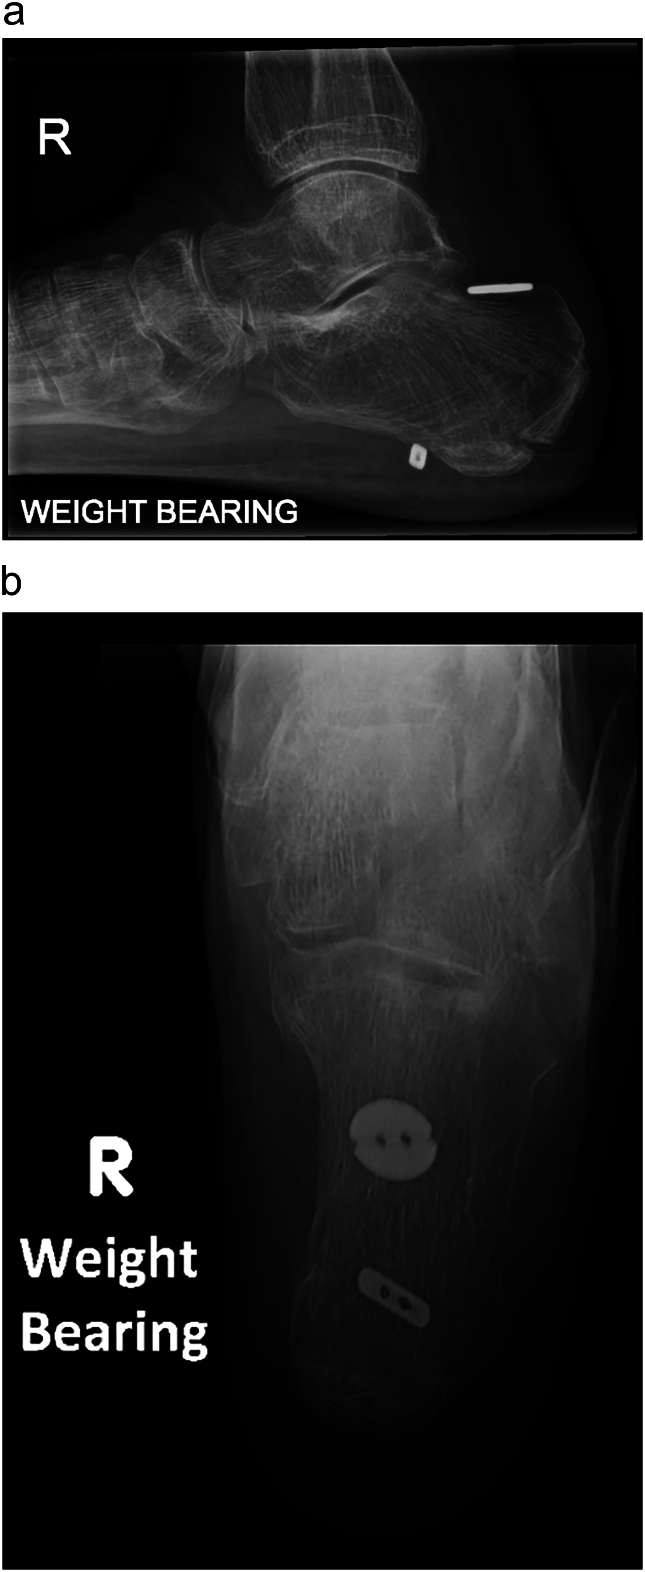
Lateral (a) and axial (b) right calcaneal radiographs at 3 months follow-up demonstrate fracture union, with no change in position of the fixation.
